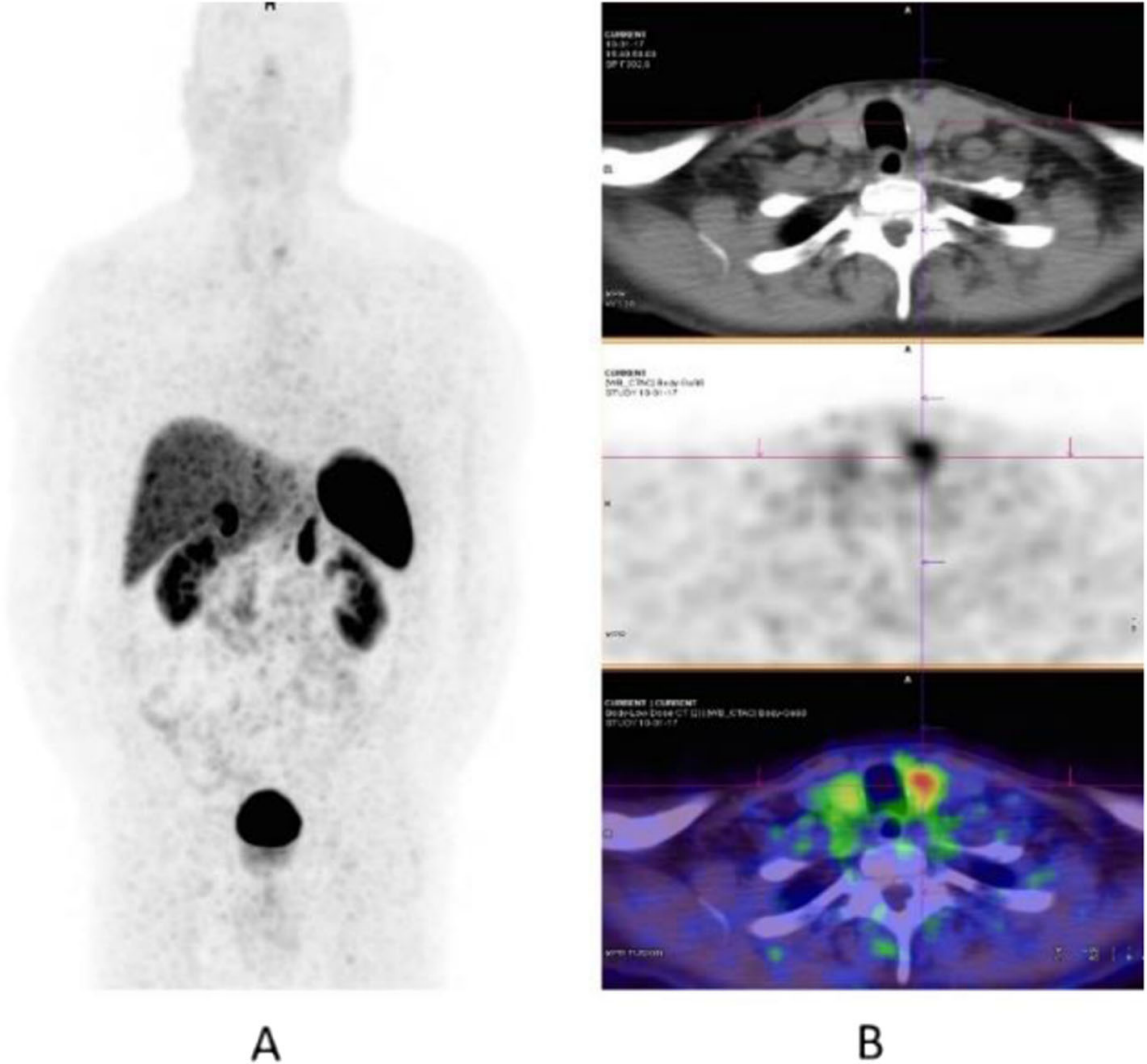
68Ga-DOTA peptide, staging medullary thyroid cancer (MTC). *Clinical history*: 70 y.o. man with newly diagnosed MTC. Suspicion of distant metastases on CT. *PET/CT findings*: moderately increased uptake in the known tumour (a MIP, b CT, PET, and fused imaging). No other suspicious lesions are seen
